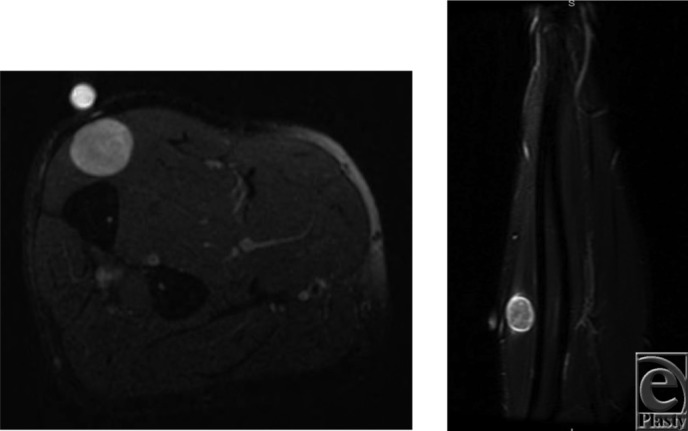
Axial T2 (a) and coronal STIR (b) MRI of the right proximal forearm with a hyperintense, well-circumscribed mass within the flexor digitorum profundus.
